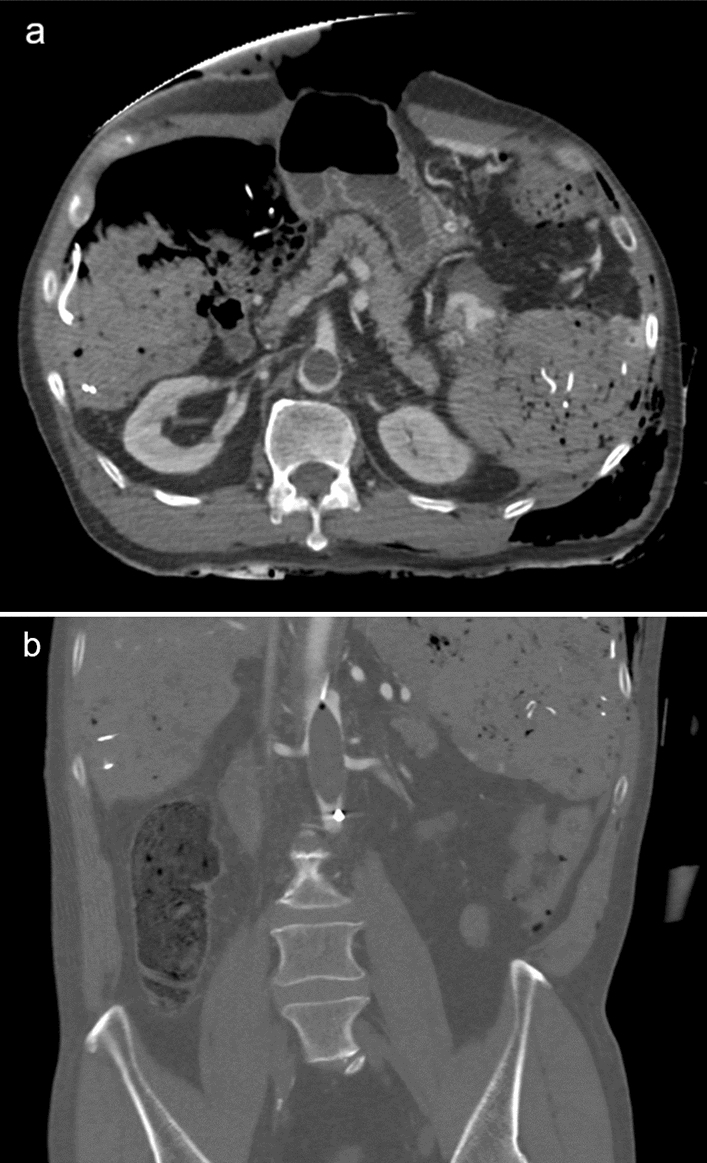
Contrast-enhancement computed tomography shows an inappropriate resuscitative endovascular balloon occlusion of the aorta for a patient with multiple trauma (a: axial, b: coronal view). The balloon was positioned at Zone II, and the stylet was not inserted in the balloon catheter
